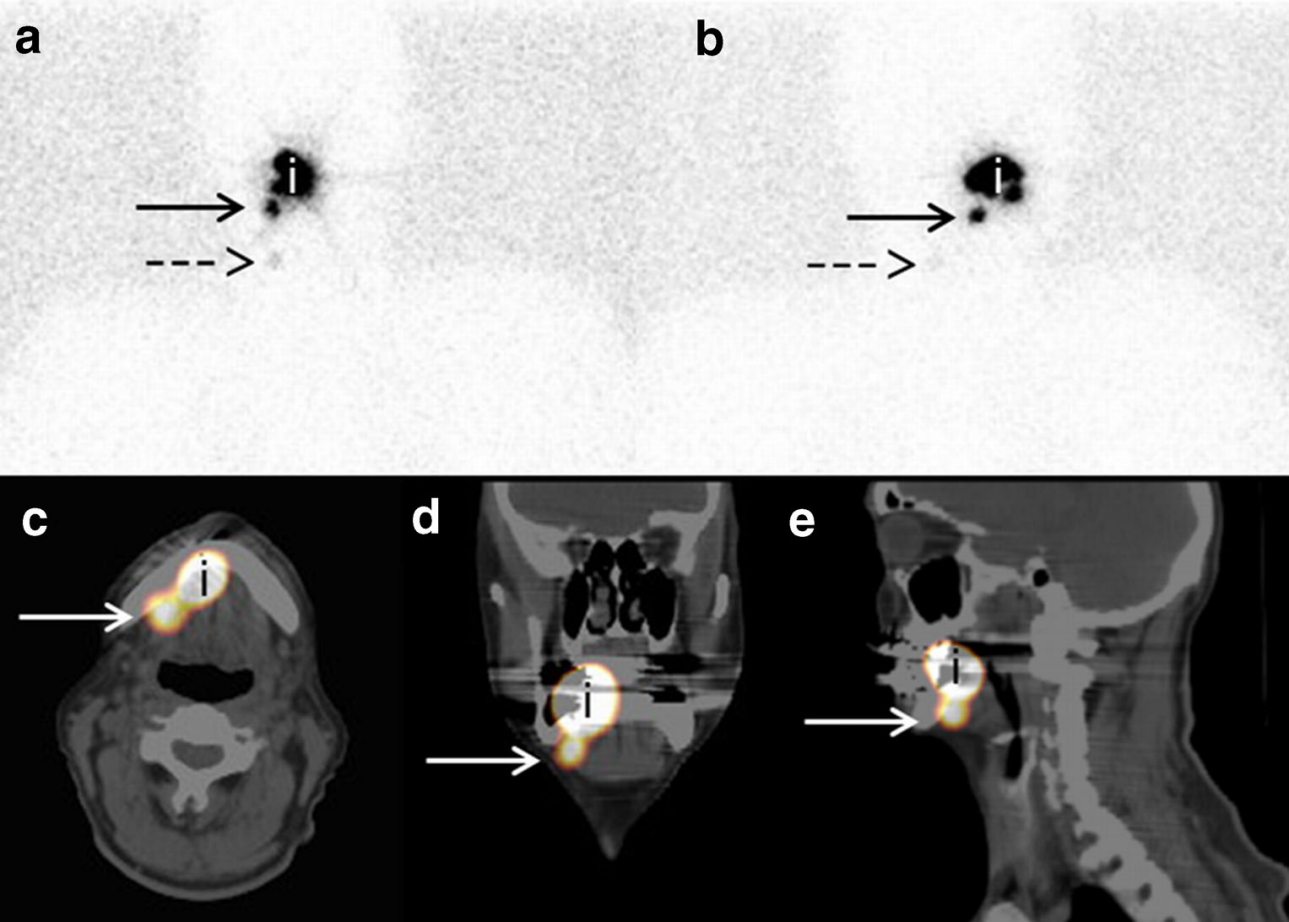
Example of better topographical orientation. Patient with a clinically T2N0 floor-of-mouth tumor on the right side. a On the planar lymphoscintigraphy, the hotspot is clearly visible (*arrow*), considered to be a sentinel lymph node in level I. Also, a less-visible hot spot was observed (*dashed arrow*), considered to be a second echelon node. b Lateral projection of the planar lymphoscintigraphy on the right side of the neck with the same intense hotspot in level I (*arrow*) and very weak uptake in the considered second echelon node (*dashed arrow*). c, d, e SPECT-CT shows a hotspot just behind the mandible (*white arrow*) with close relationship to the injection spot of the primary tumor (*i*) and was actually considered as a sublingual node
